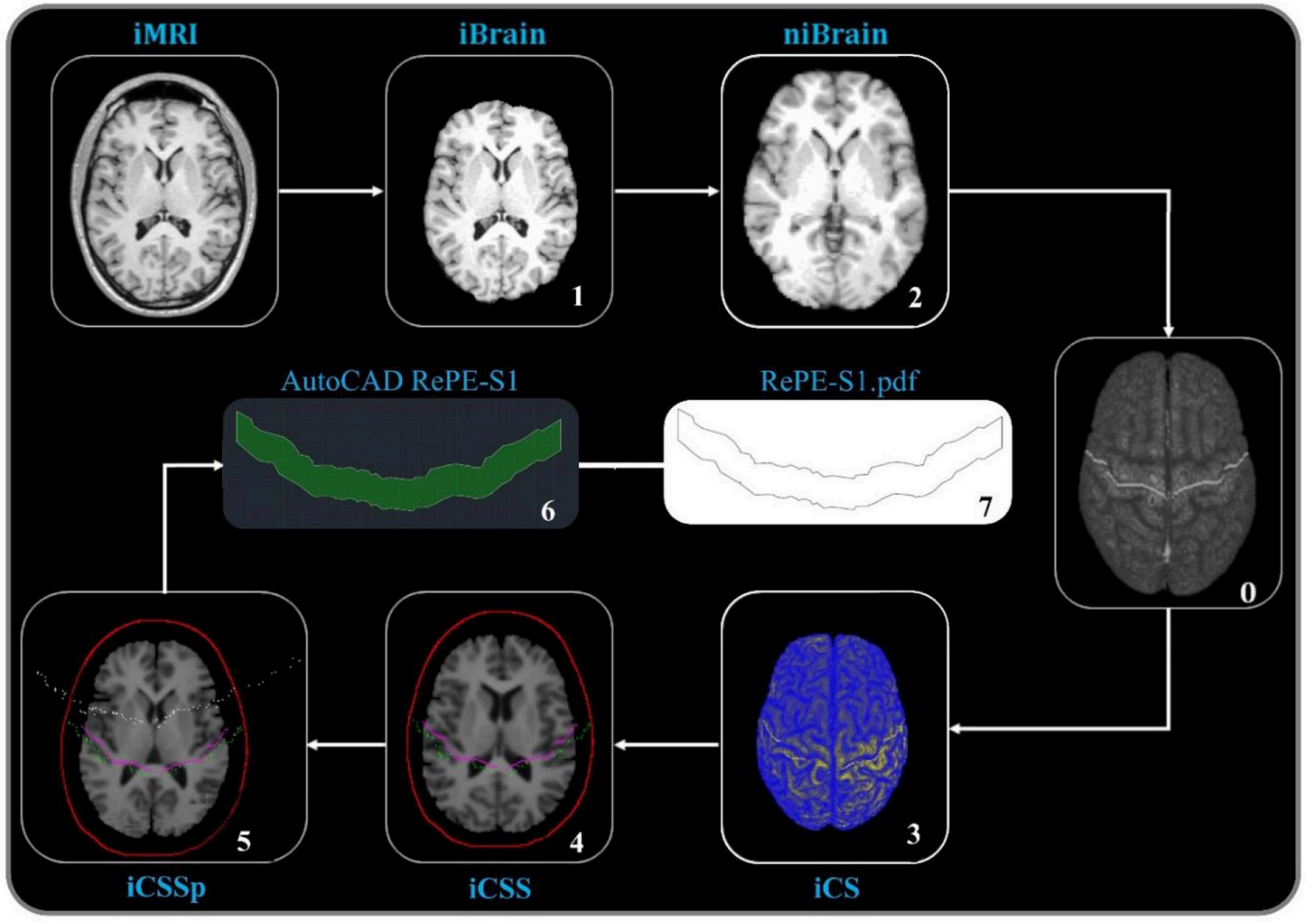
Computerized procedure to shape RePE. As detailed in the Methods, the steps from the individual brain MRI (iMRI), with segmentation of the brain (iBrain, 1), transformed into MNI standard model (niBrain, 2), where the central sulcus identified points are stored (once for every person, thus no number in the process applied in individual subject, 0) and retro projected obtaining the individual central sulcus (iCS, 3), projected on the scalp (iCSS, 4), and further projected on a planar surface (iCSSp, 5) so that by AutoCAD the RePE area is obtained (6) and printed (7).
